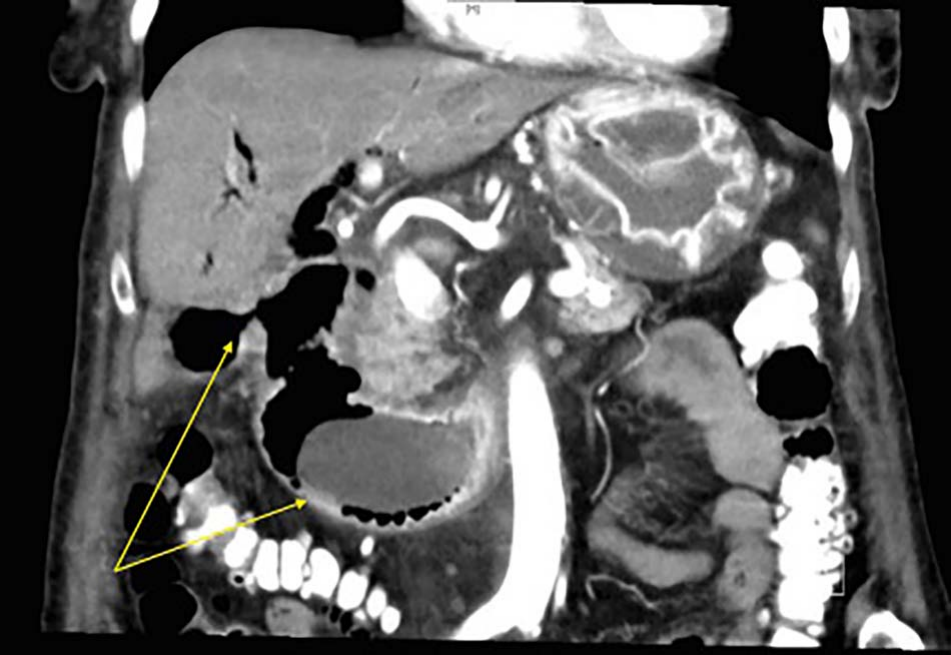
Coronal CT image of abdomen with arrows demonstrating a fistulous connection between the gallbladder and duodenum, and a large gallstone in the second part of the duodenum.
